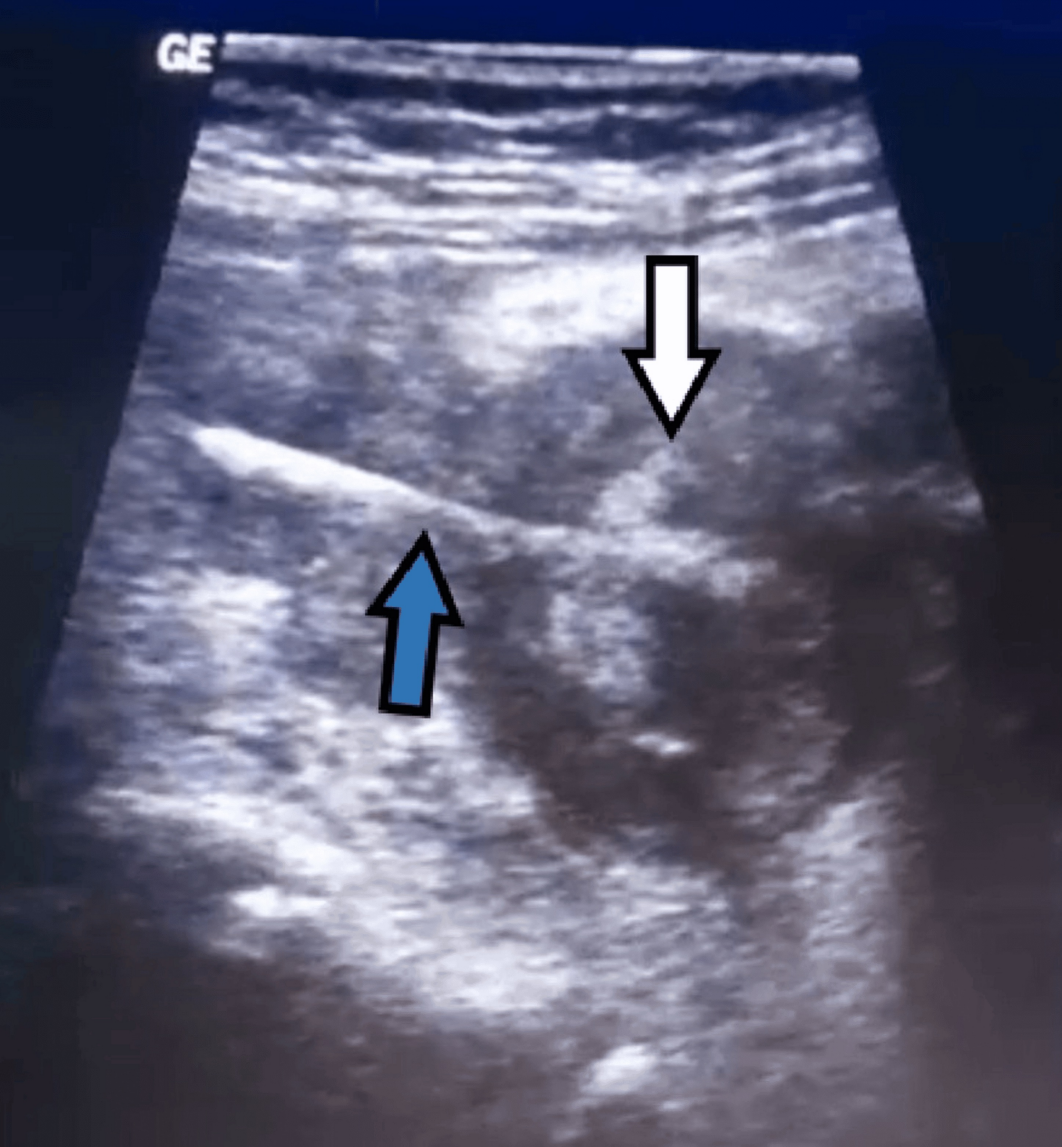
Ultrasound image showing a pin-shaped foreign object (blue arrow) located in the appendix (white arrow)
